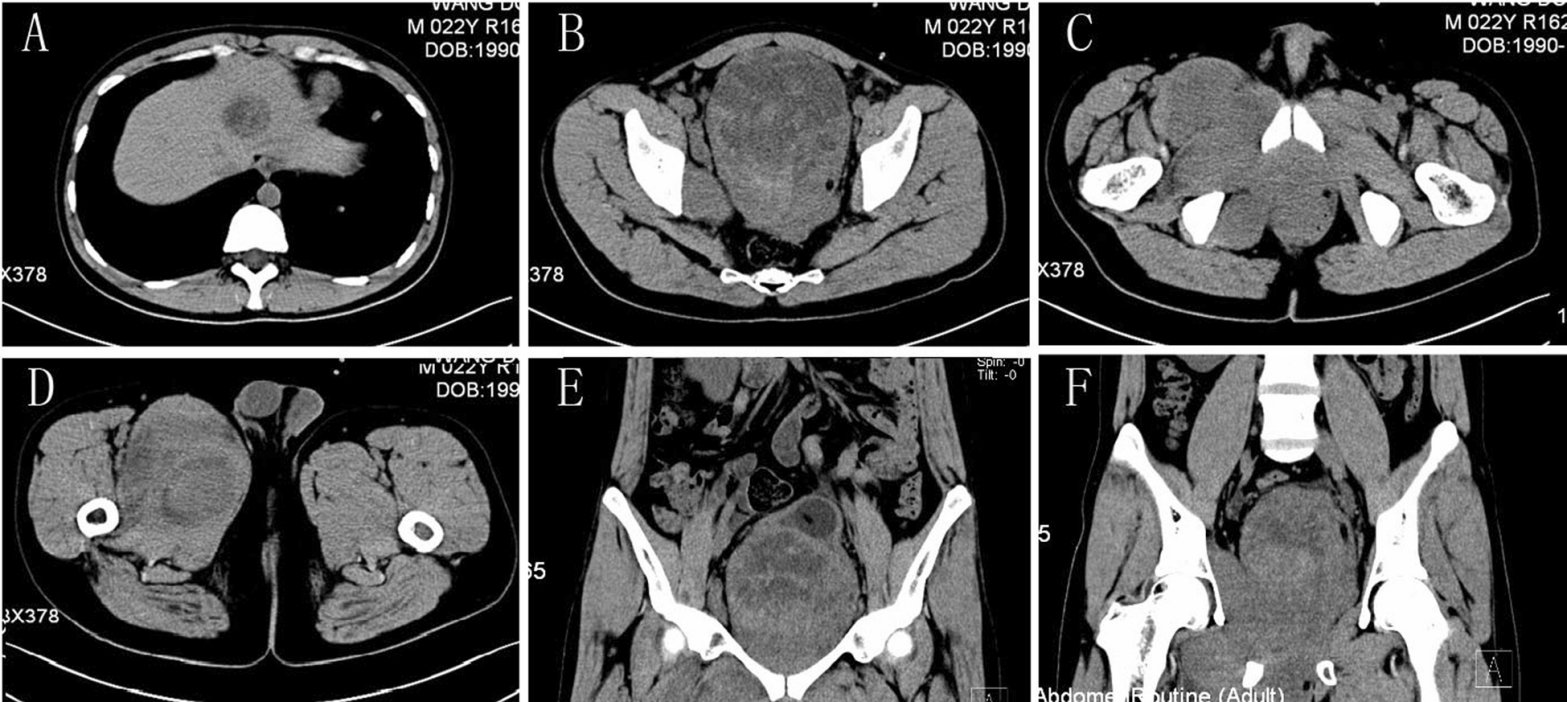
Enhanced pelvic computed tomography. (A) Evidence of liver metastasis. (B-F), Enhanced pelvic computed tomography revealed a 14-cm mass that appeared to originate in the prostatic fascia. An 8.5-cm mass was found on pelvic magnetic resonance imaging.
